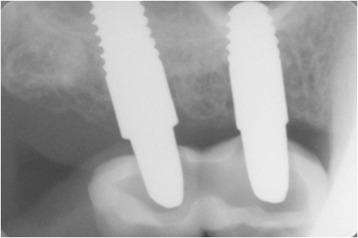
Periapical X ray after 1 year of follow-up, the bone was stable and no sign of peri-implantitis was shown
